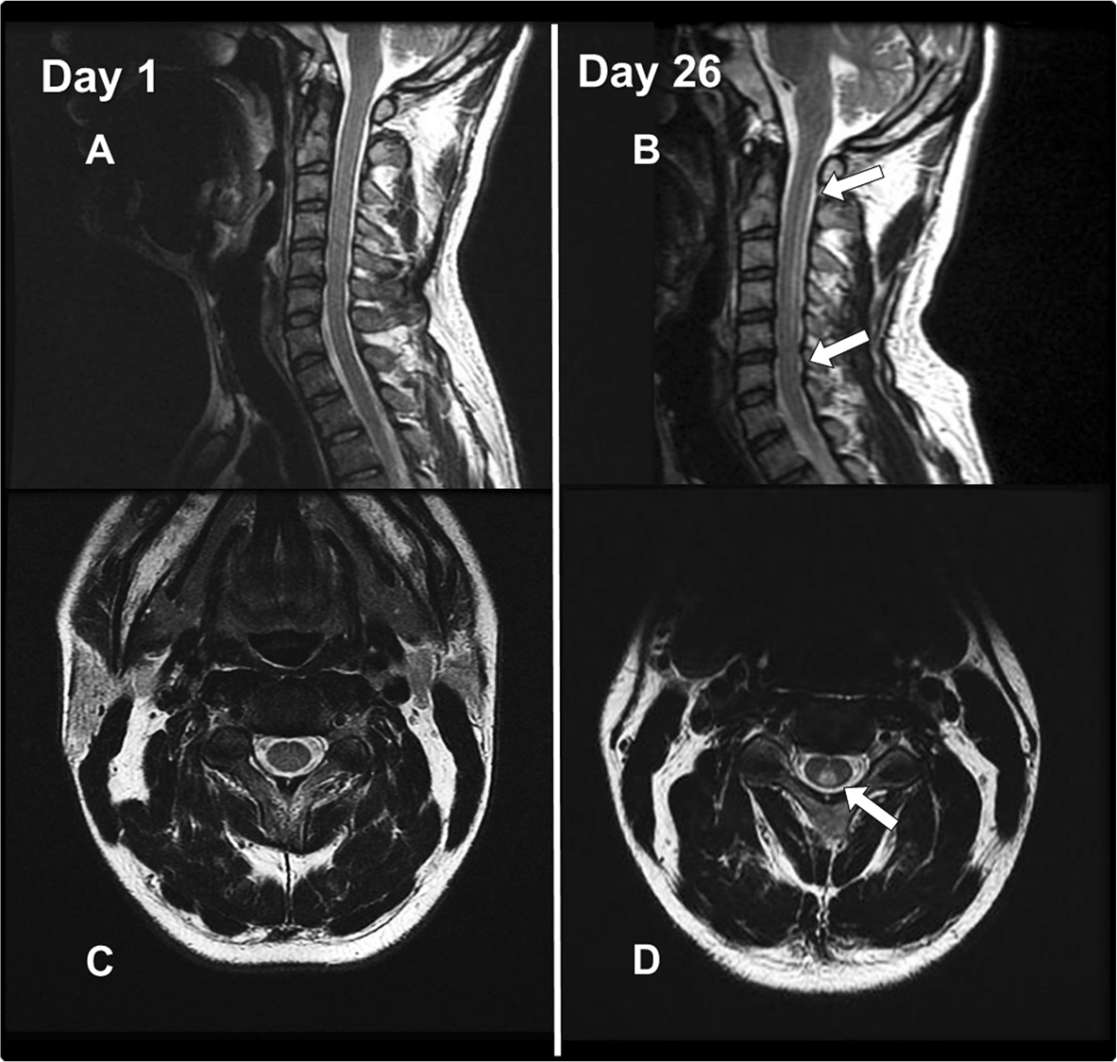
T2-weighted MR images of the spinal cord on day 1 and day 26 after admission. On day 26, hyperintense lesions are present at cervical C2–C6 levels (B, *arrows*). White matter hyperintensities are also observed on T2-weighted MR images in the posterior portion of the spinal cord (d)
